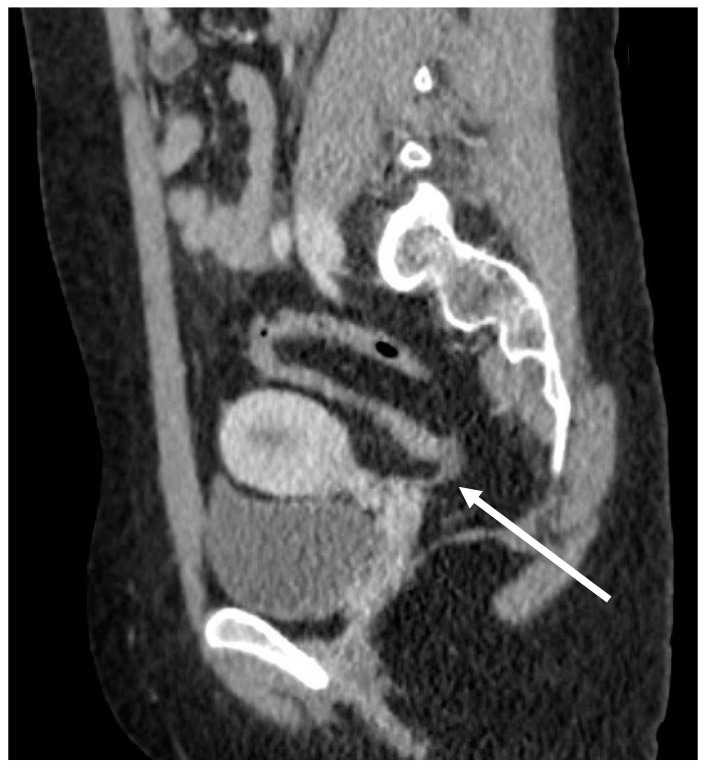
Sagittal view of an abdominal CT scan from admission showing sigmoid colon narrowing (arrow).
